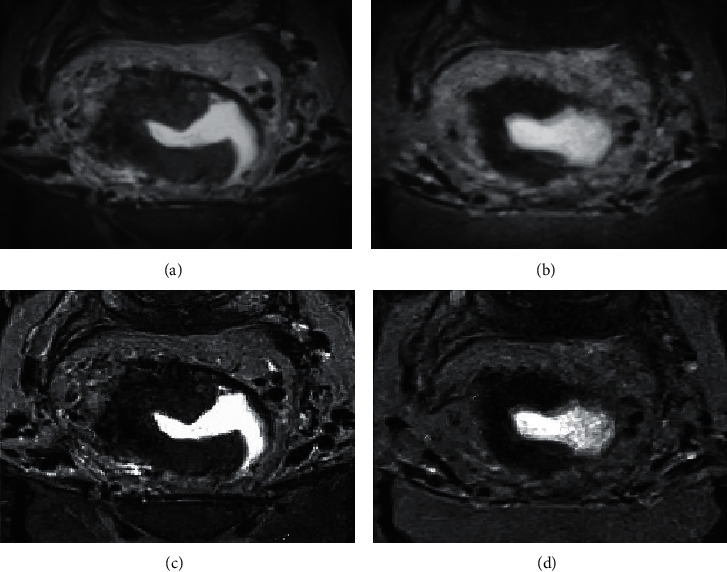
Comparison of conventional MRI and optimized MRI before and after NCRT. (a) Conventional MRI image before NCRT treatment; (b) conventional MRI image after NCRT treatment; (c) optimized MRI image before NCRT treatment; and (d) optimized MRI image after NCRT treatment.
